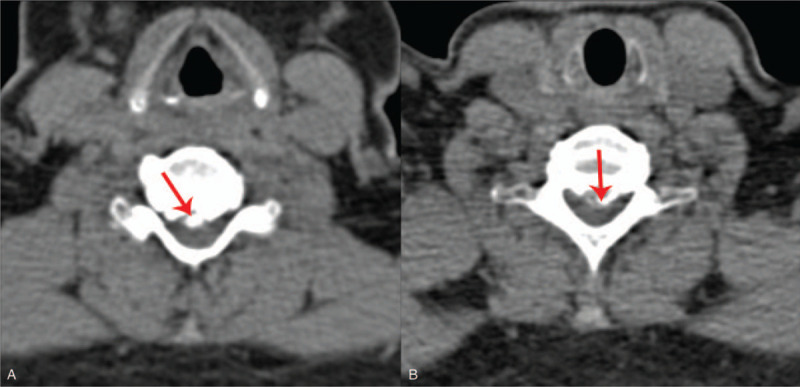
CT images revealed deflation of physiological curvature of the cervical spine, hyperosteogenesis of multiple cervical vertebrae, and strip-shaped high-density shadows with spinal stenosis in the C5-C7 segment (red arrow).
